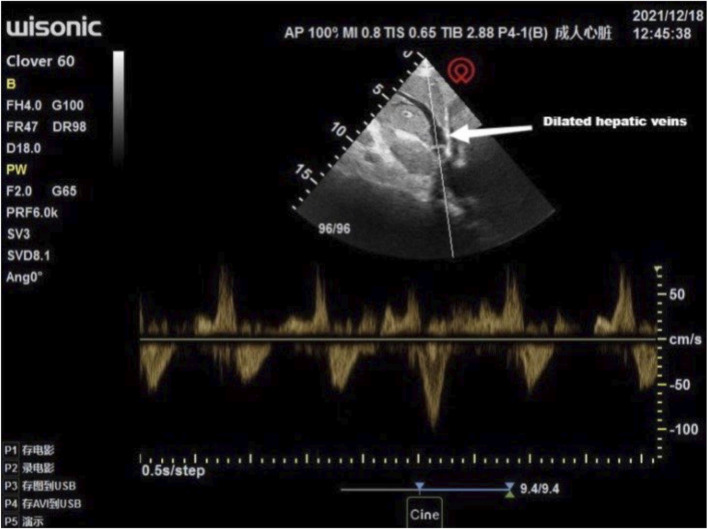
Transthoracic echocardiography showed a dilated hepatic vein.
